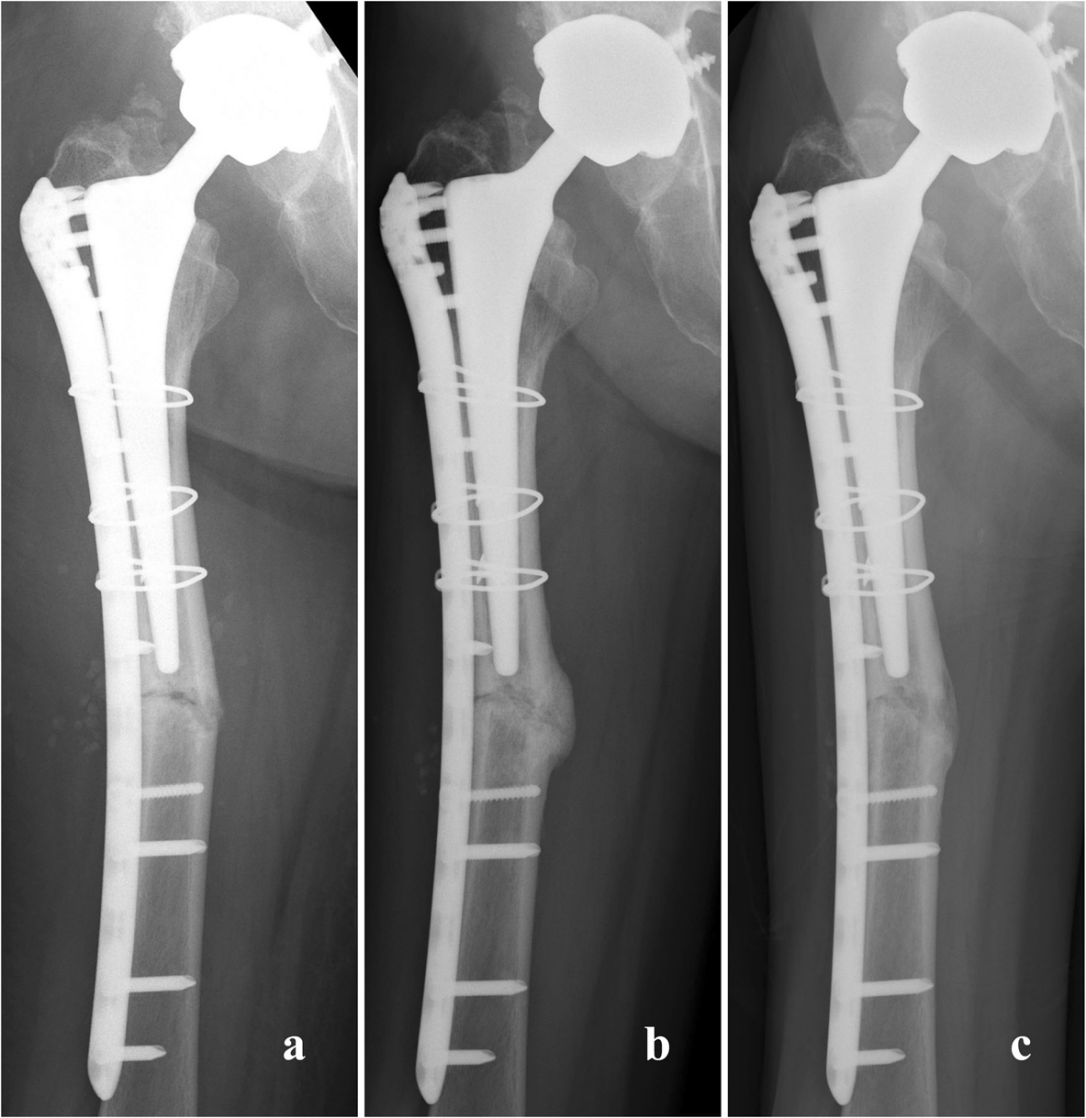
Radiographs obtained after osteosynthesis. (a) Just after the surgery. (b) At 6 months after surgery. (c) At 2 years after surgery.
